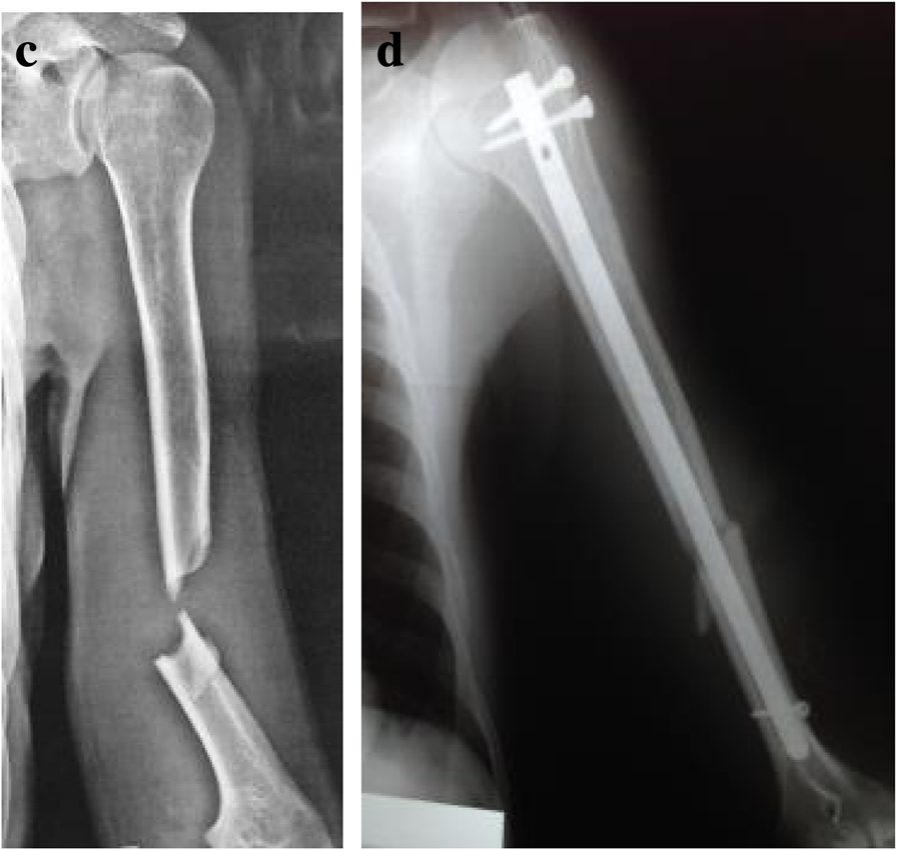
Radiographies of the left arm (c) of a 40 year-old patient with diaphyseal humerus fracture treated with long-locked intramedulary nailing (d), with implant placement through the rotator cuff
